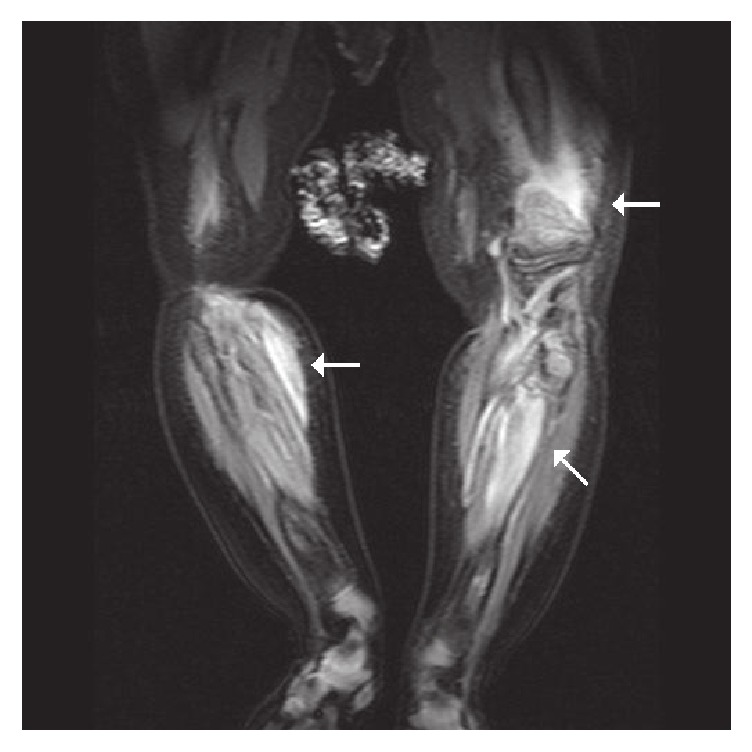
MRI of both lower legs: the T2-weighted coronal images show metaphyseal fractures involving distal left femur and proximal bilateral tibias with extensive periosteal hemorrhage or edema, favored child abuse.
